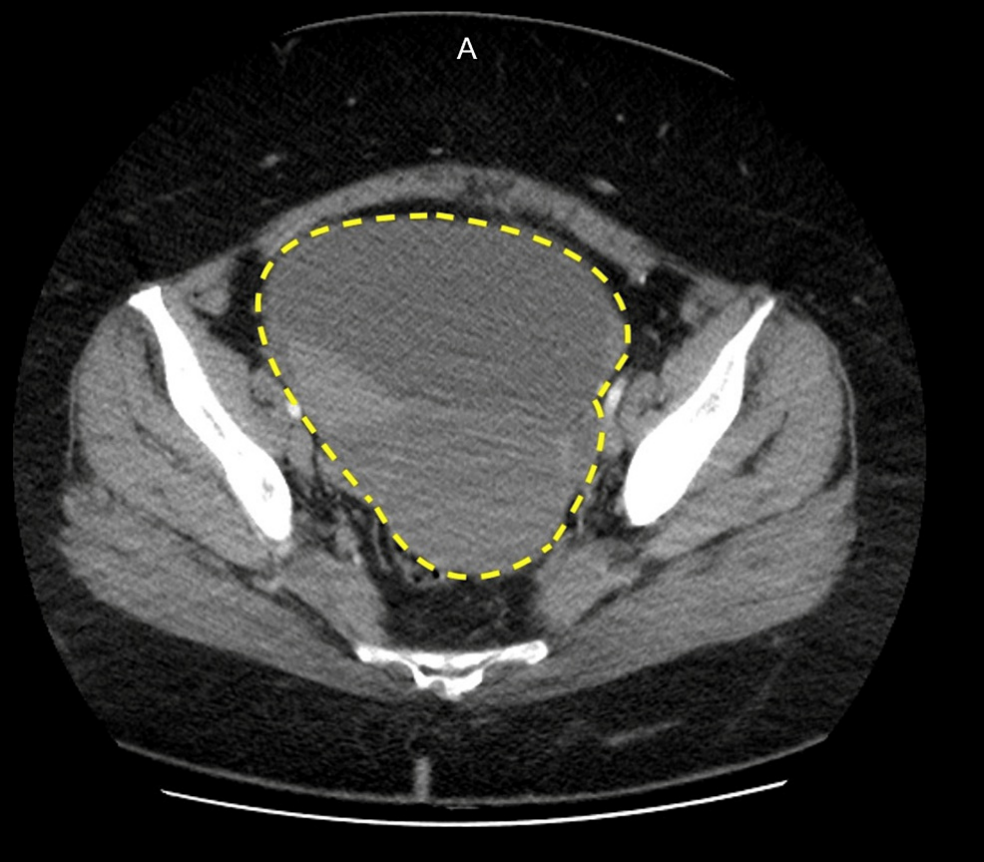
Transverse contrast-enhanced abdominal and pelvic CT showing a large cystic pelvic mass, outlined by a dotted yellow line.
